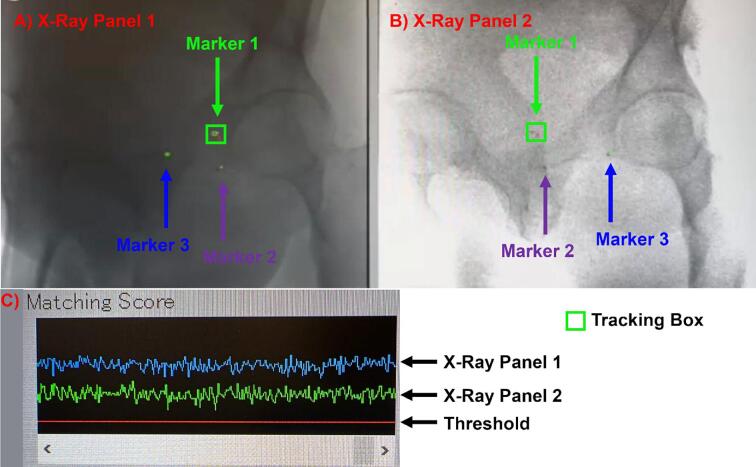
A) X-ray Panel 1. 1B) X-ray Panel 2. C) Matching score of both X-ray panels (Green − X-ray Panel 1, Blue − X-ray Panel 2) on Marker 1. The red line depicts the threshold of 30. (For interpretation of the references to colour in this figure legend, the reader is referred to the web version of this article.)
